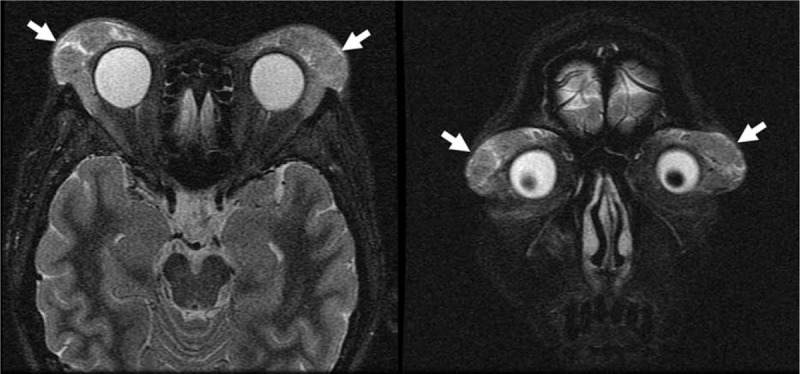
Cranial magnetic resonance imaging (MRI) revealed bilateral lacrimal gland enlargement (arrows).
